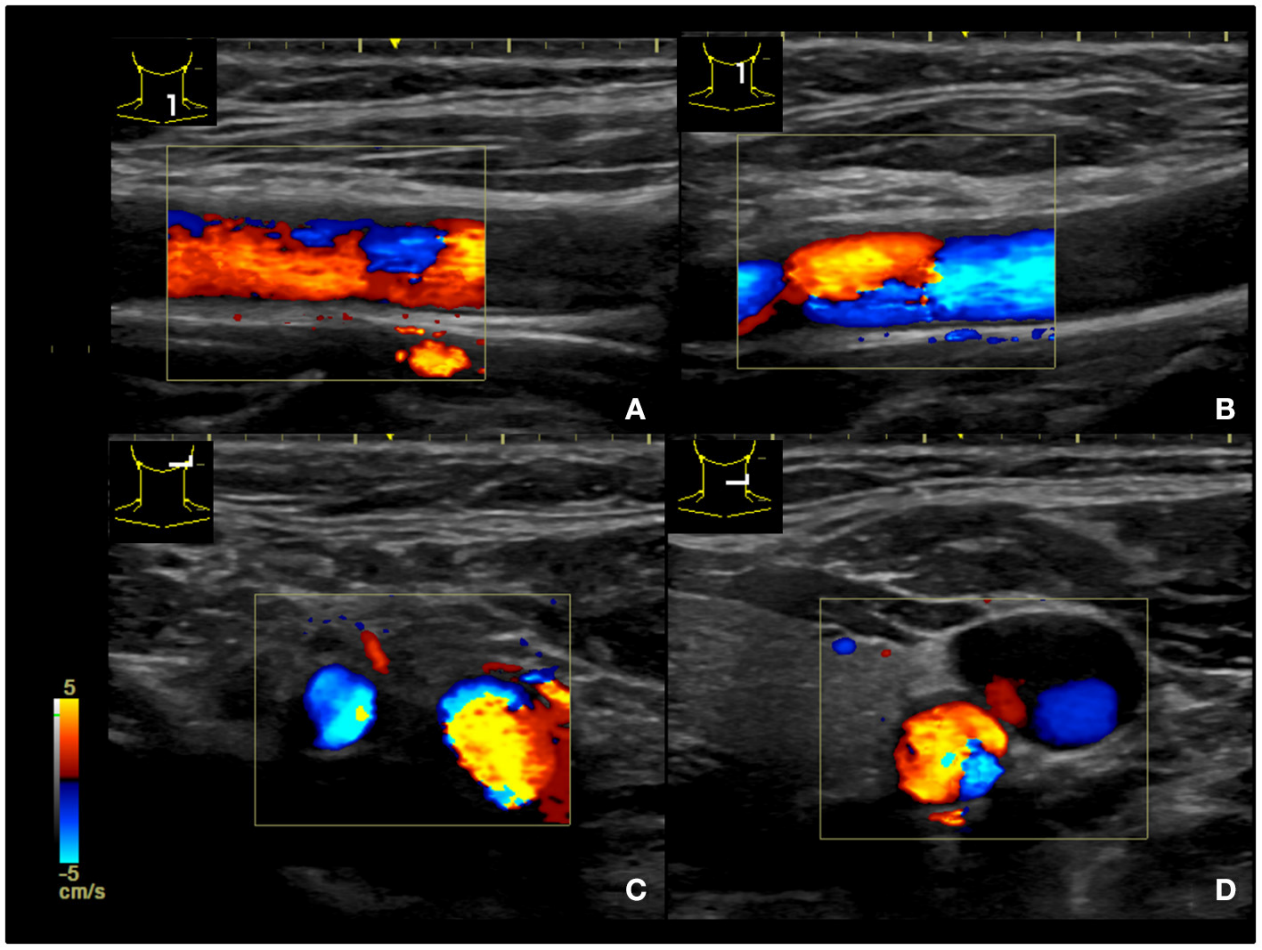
Ultrasound survey of the carotid artery revealing intact structures. (A,B) longitudinal plane of the carotid artery. (C,D) transverse plane of the carotid artery.
